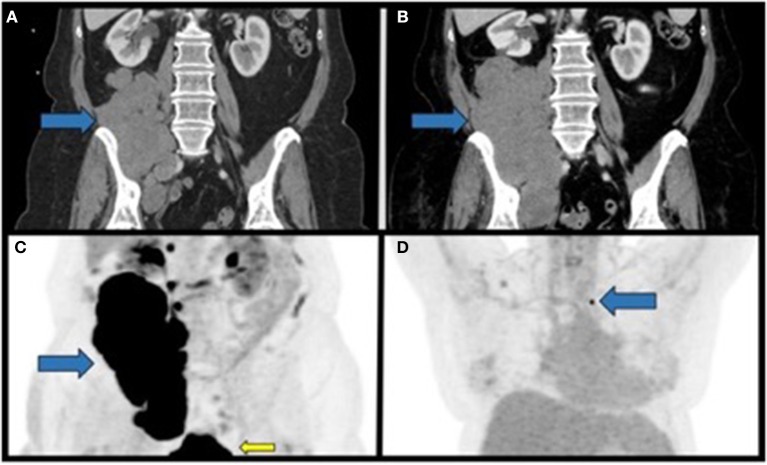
Staging CT scans and PET imaging of the primary tumor and metastatic lesion prior to radiation therapy. (A) Coronal section of the initial CT of the abdomen/pelvis with contrast identifying a large soft-tissue mass in the right retroperitoneal space overlying the right psoas muscle with extension into the right hemi-pelvis. (B) Coronal section of a CT of the abdomen/pelvis approximately 2 months after the initial diagnosis demonstrating interval growth of the now inoperable retroperitoneal mass. (C) Coronal PET/CT maximum intensity projection (MIP) performed approximately 3 months after initial diagnosis, demonstrating the large FDG-avid mass in the right retroperitoneal space (blue arrow). FDG-avidity in the inferior aspect of the image in the figure corresponds to physiological uptake in the urinary bladder (yellow arrow). (D) Coronal PET/CT MIP from the same study as in C demonstrating a non-enlarged FDG-avid lymph node in the left supraclavicular nodal station. Blue arrows, primary mass; yellow arrow, physiologic FDG uptake.
